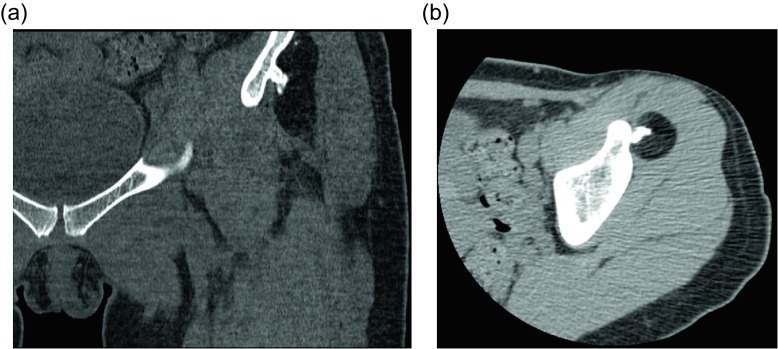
Parosteal lipoma: 45-year-old woman with history of left hip “osteochondroma”. Coronal CT image viewed with bone windows (a) and axial CT image with soft tissue windows (b) show a fat-attenuating lesion within the deep soft tissues of the left thigh. The lesion is intimately associated with the underlying iliac bone and an associated irregular, ossific protuberance arising from the iliac bone.
